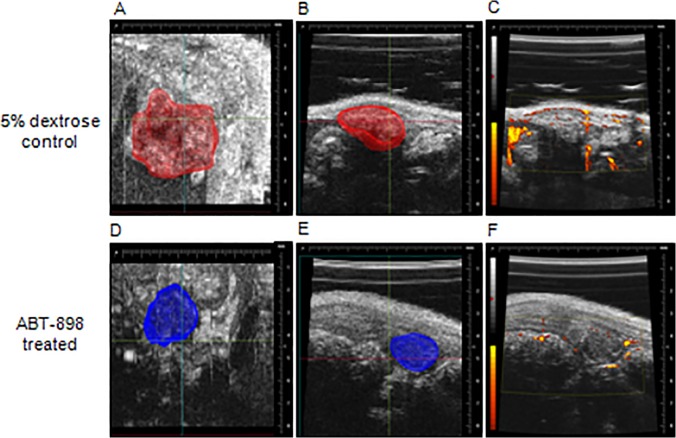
Ultrasound images of human endometriotic lesions.
